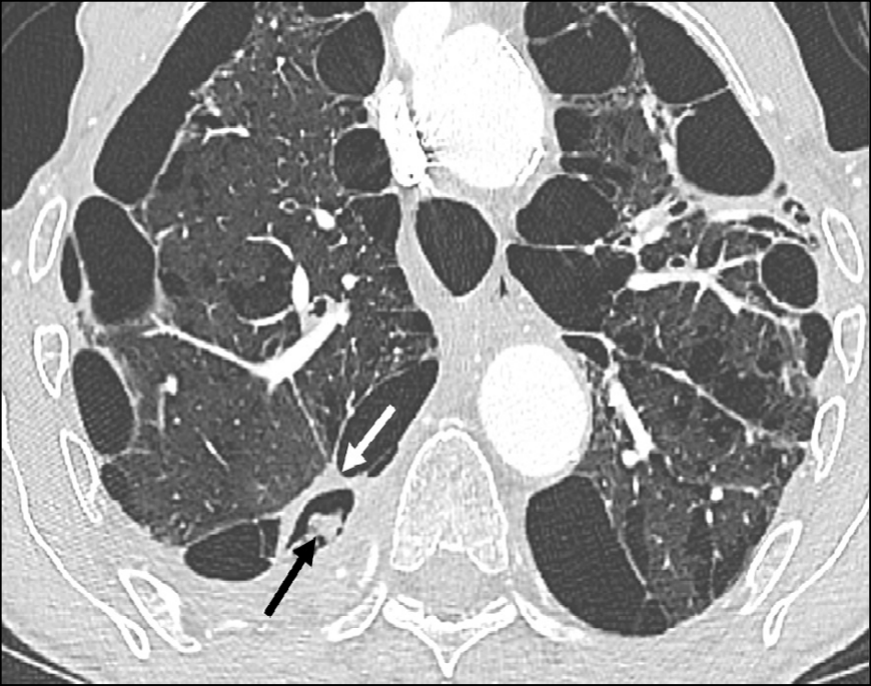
Axial CT section in the parenchymal window shows thickening (white arrow) of the wall of an emphysema bulla with endocavitary material (black arrow).
